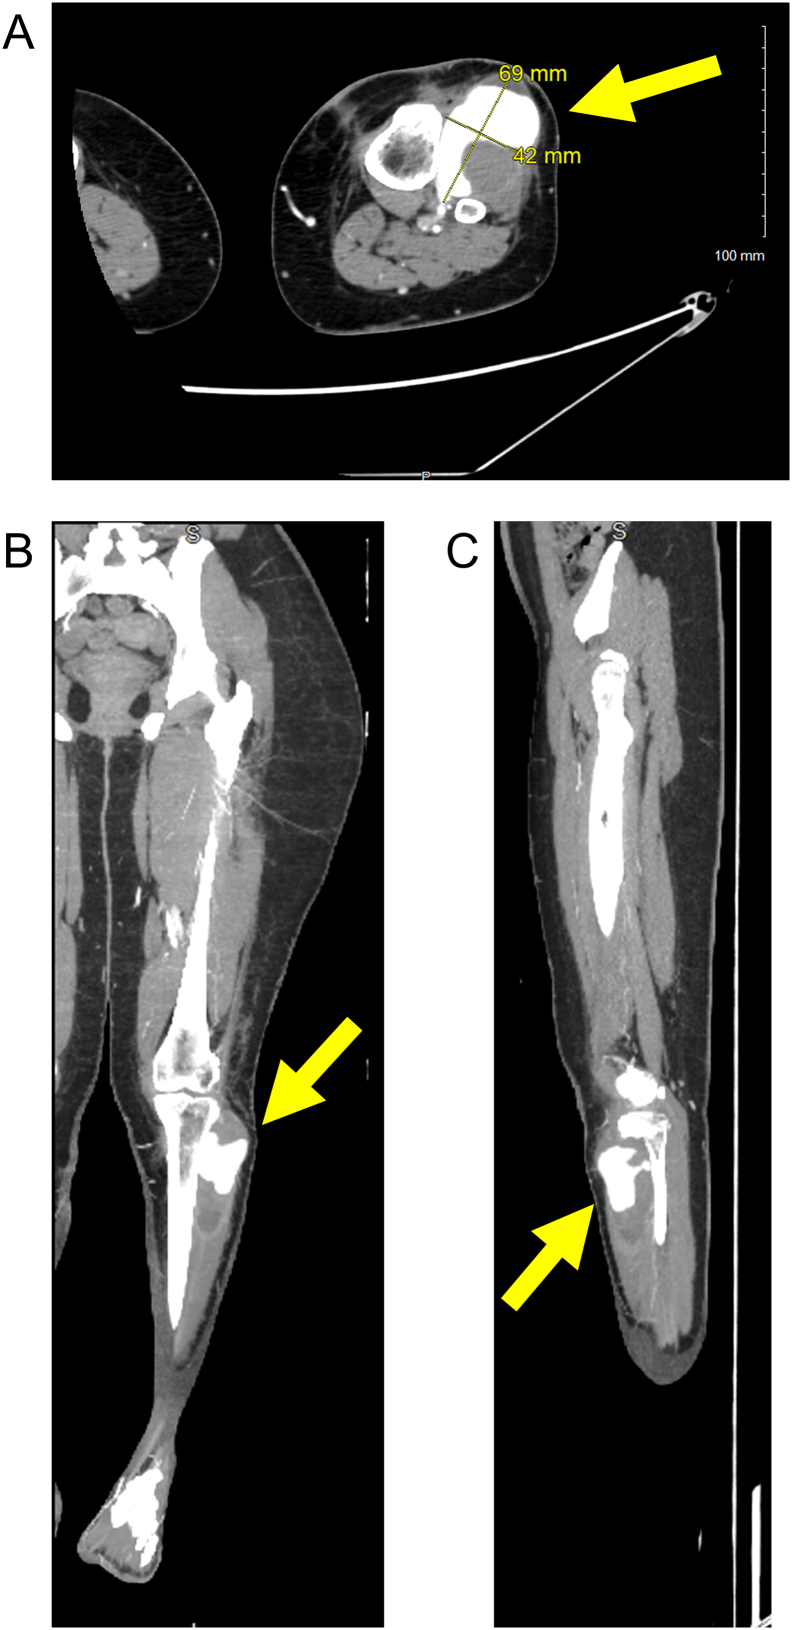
Computed tomography angiography of the left lower extremity demonstrating a large complex pseudoaneurysm arising from the anterior tibial artery, indicated with yellow arrows. A) Axial view, B) coronal view, and C) sagittal view.
